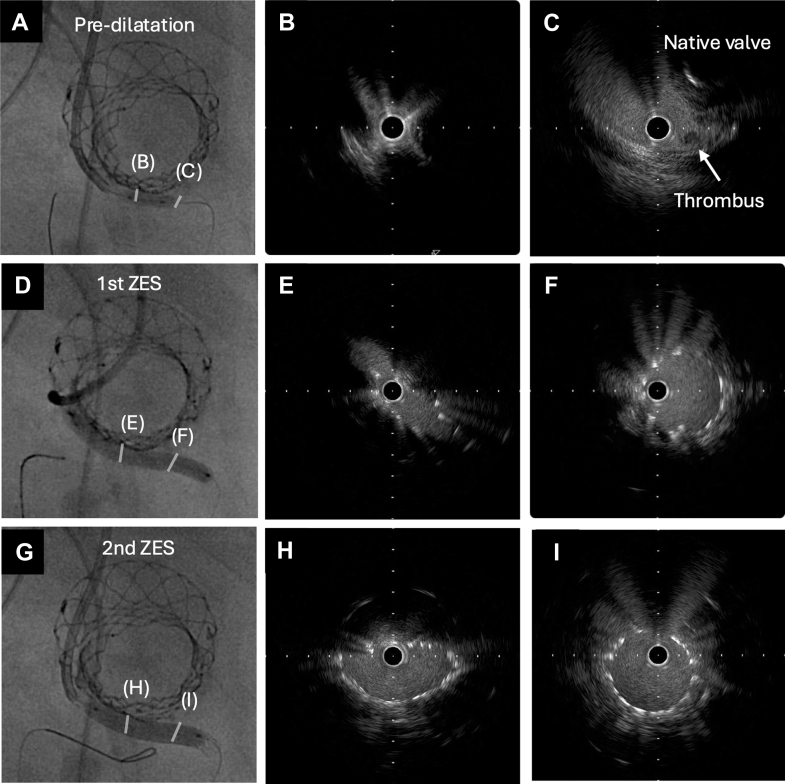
Intravascular Ultrasound Before and After Stent Implantation (A to C) IVUS demonstrating the presence of a thrombus and native valve tissue within the right coronary sinus. (D to F) After implantation of the first zotarolimus-eluting stent (ZES), IVUS showed compression of the stent by the transcatheter heart valve. (G to I) Subsequent IVUS imaging after the placement of a second overlapping ZES and postdilation with a 5.0-mm noncompliant balloon confirmed the restoration of an acceptable luminal area. IVUS = intravascular ultrasound.
